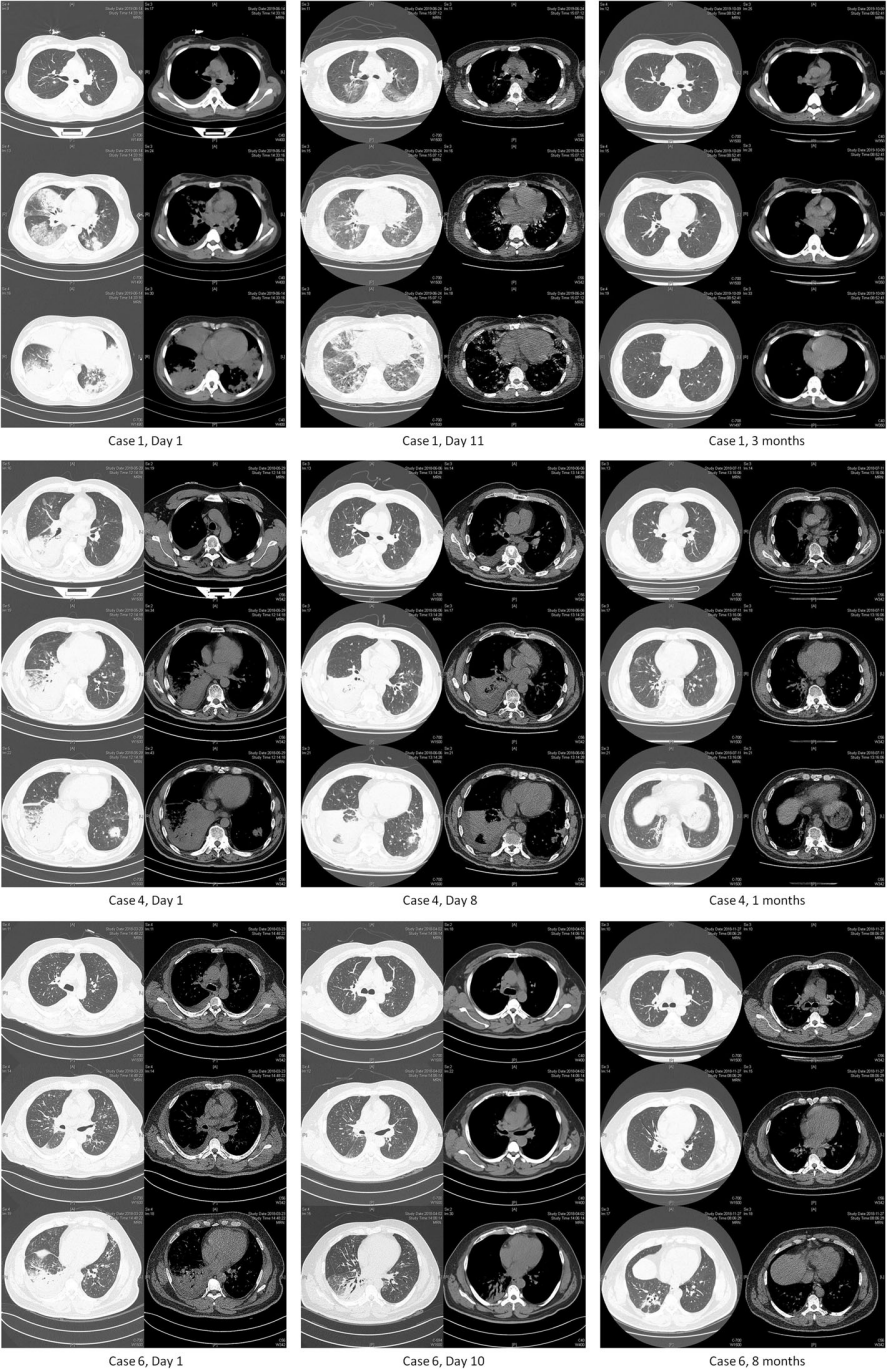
Chest computed tomography (CT) on day 1, day 8–11, followed up after 1 months to 8 months of three patients (case1, case 4 and case 6). Three representative slices of the upper, middle and lower lobe were chosen
